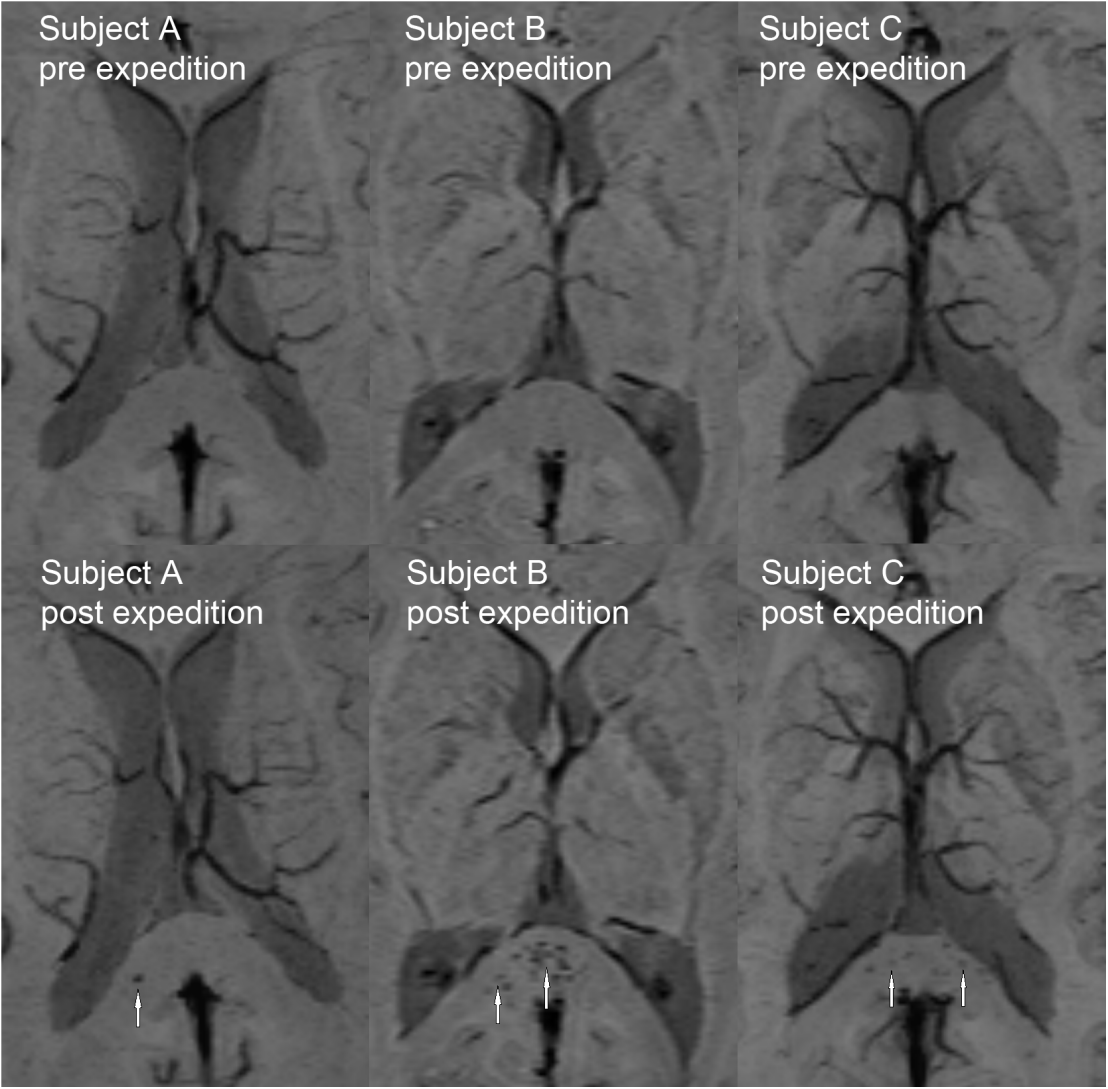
Imaging findings pre- and post-expedition in subjects with new microhemorrhages after the climb. Subject A: Comparison of SWI (MinIP) in pre- and post-expedition MRI shows a new solitary microhemorrhage (indicated by arrow). SWI of Subject B and SWI (MinIP) of subject C show multiple new microhemorrhages of the corpus callosum visible in post-expedition MRI (arrows).
